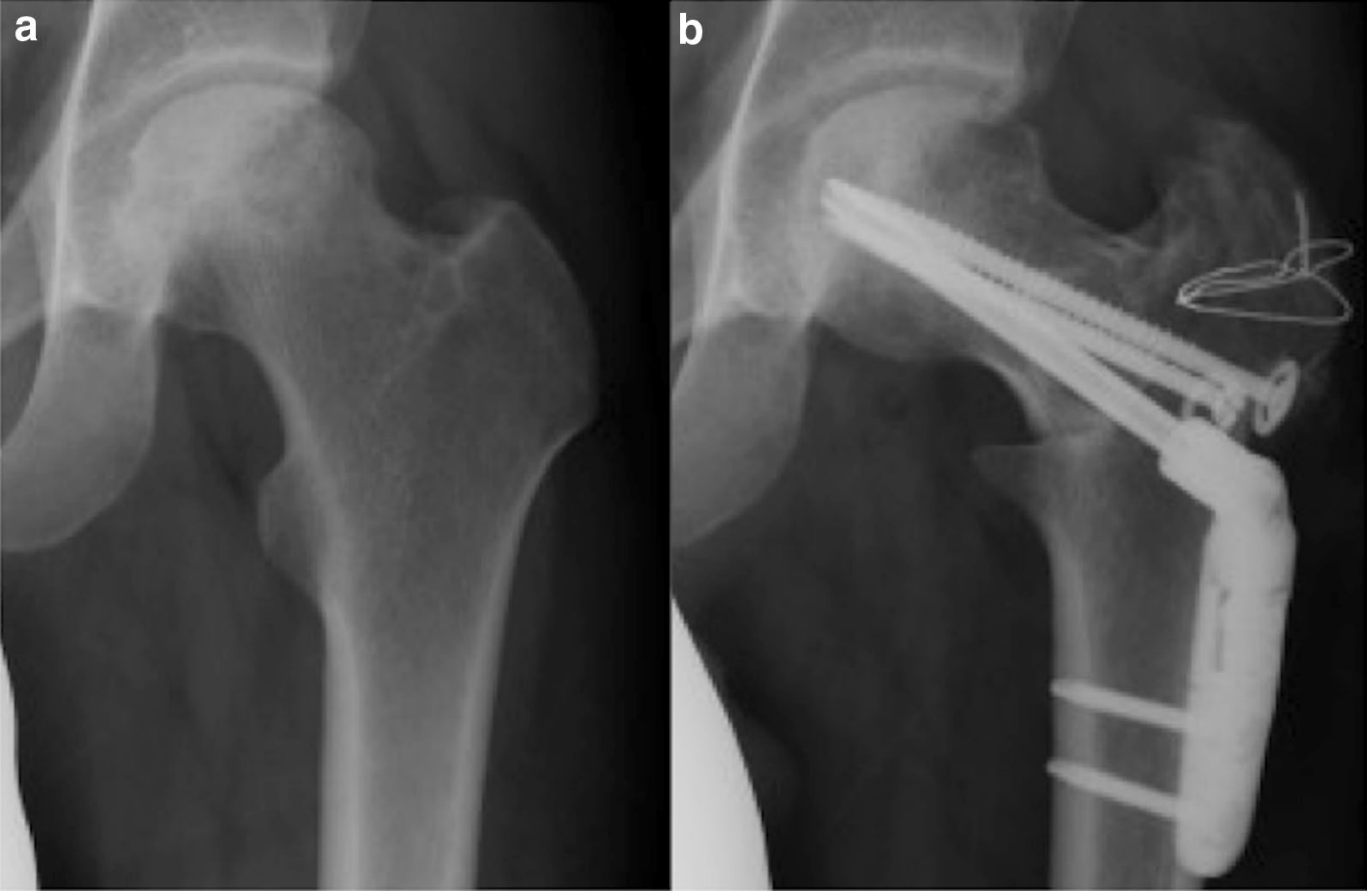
A 28-year-old male with unilateral ONFH treated with ARO (Patient 5). a Preoperative anteroposterior radiograph shows left femoral head collapse and bone sclerosis, which indicates ONFH with stage 3A and type C1. b Anteroposterior radiograph after ARO of the left hip
